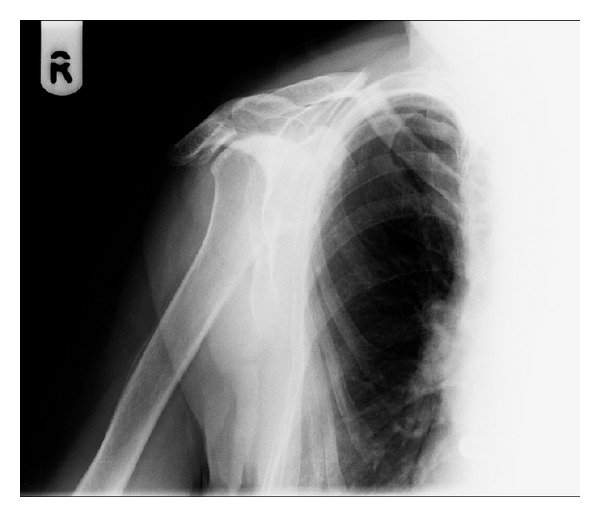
Radiograph taken in A&E department, demonstrating acute transverse right mid-clavicular fracture.
